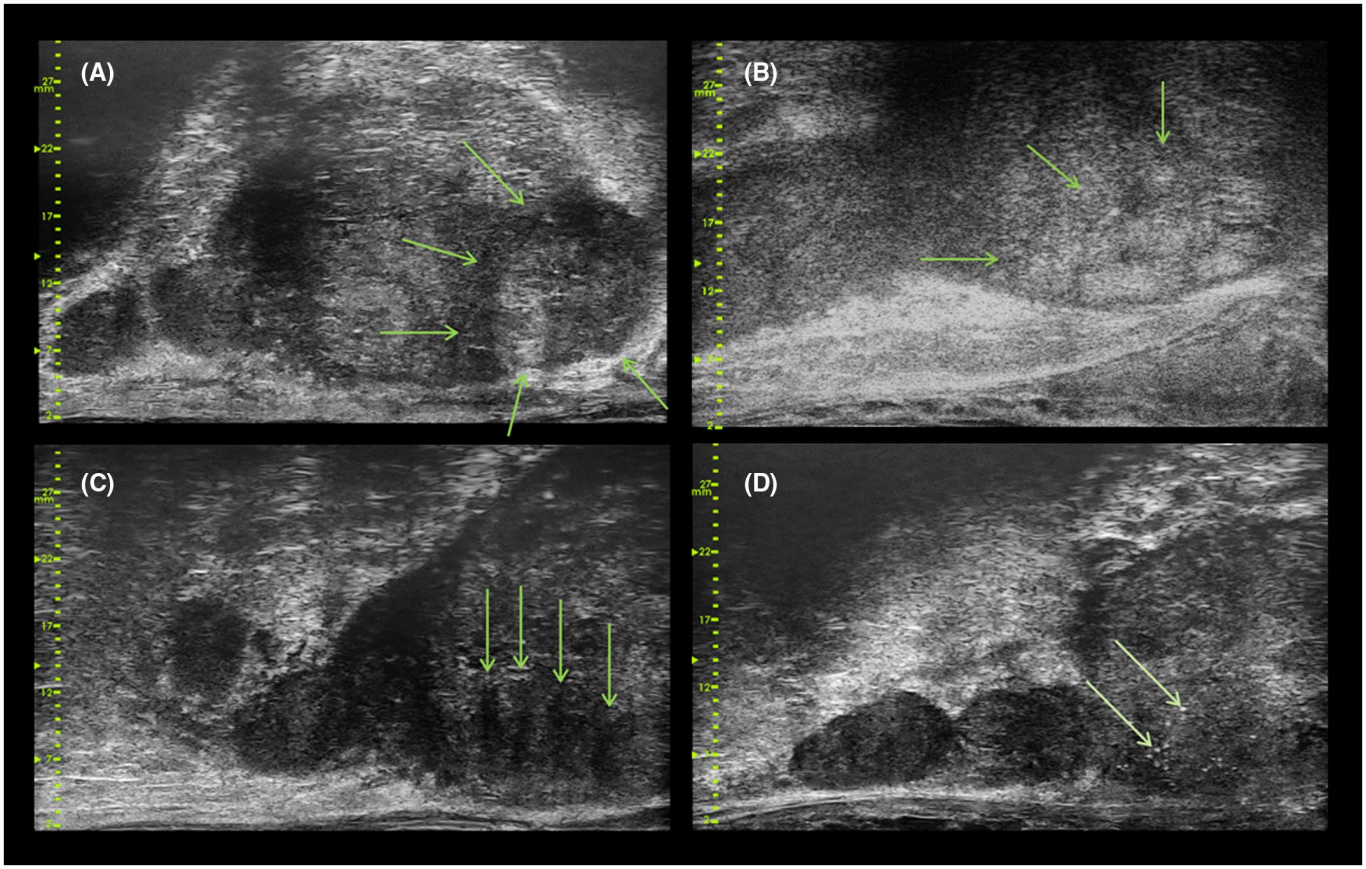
Examples of micro‐ultrasound guided biopsy targets. (A) Apex lateral mixed‐echo PRI‐MUS 5 lesion. Biopsy of this area confirmed Gleason Sum 7 (4+3) cancer in 80% of the core. (B) Mid‐prostate posterior PRI‐MUS 4 “cauliflower” patterned lesion. Biopsy of this area confirmed Gleason Sum 8 disease. (C) Base PRI‐MUS 5 irregular shadowing confirmed as Gleason Sum 7 (4+3) cancer on biopsy with 95% core involvement. (D) Base PRI‐MUS 4 bright echo pattern confirmed as Gleason Sum 7 (3+4) disease
